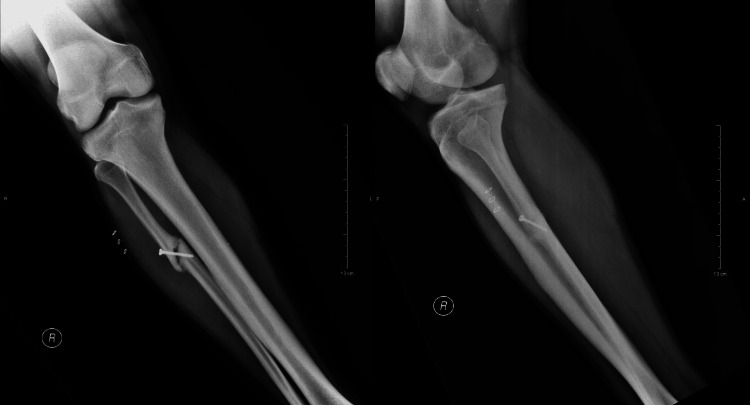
Postoperative radiographs depicting fixation with a lag screw
